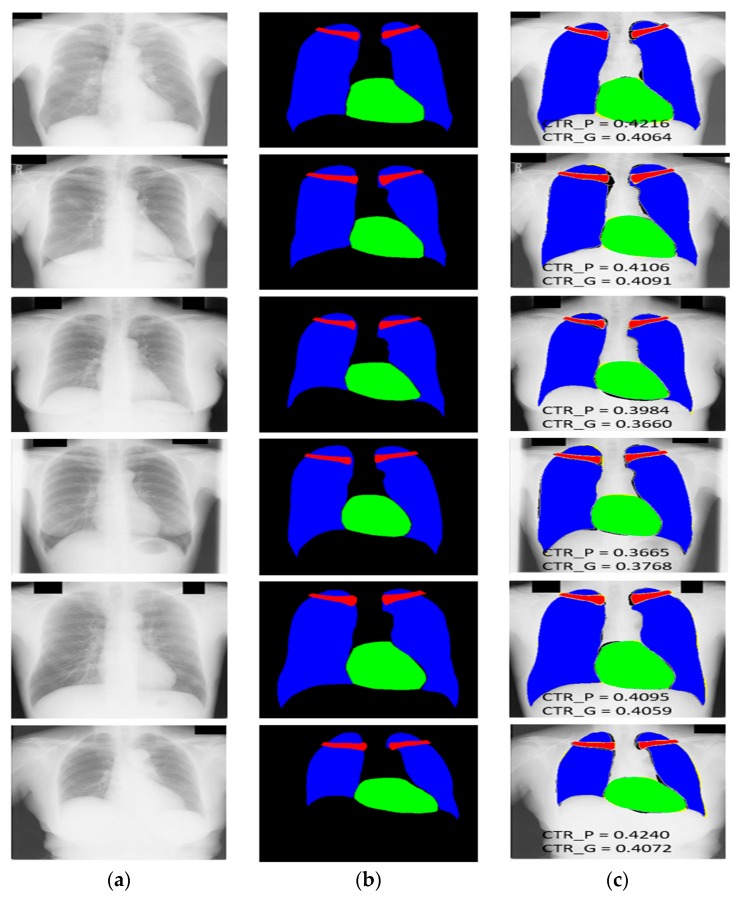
Examples of chest anatomical structure segmentation by X-RayNet for the JSRT dataset: (a) original CXR image; (b) ground-truth mask; (c) predicted mask result by X-RayNet; false positives (*FP*) (shown in black for each class), false negatives (*FN*) (shown in yellow for each class), and true positives (*TP*) (shown in blue, green, and red for the lung, heart, and clavicle bone classes, respectively). CTR_P and CTR_G represent the CTR predicted by the proposed method and CTR by ground-truth mask.
